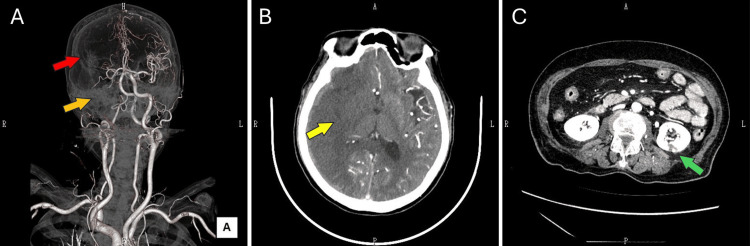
Contrast-enhanced CT image obtained three hours after the ICU administration A: 3D reconstructed CT Angiography image. B: Axial contrast-enhanced CT image of the head at the level of the anterior and posterior horns of the lateral ventricles. C: Axial contrast-enhanced CT image of the abdomen at the level of the kidneys. In A, contrast filling defects are observed in the right internal carotid artery (orange arrow) and the right middle cerebral artery (red arrow). In B, a large area of contrast defect and hypodensity is noted in the right middle cerebral artery territory (yellow arrow). In C, a partial infarction of the left kidney is observed (green arrow).
